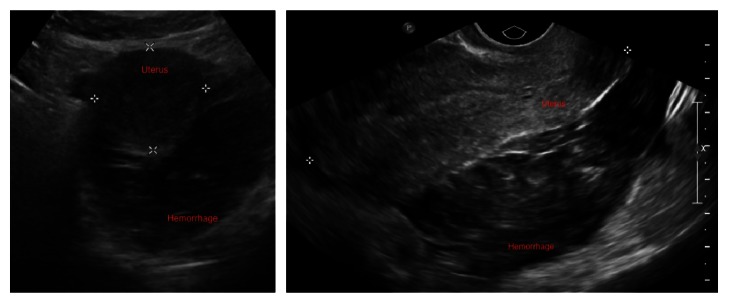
Transverse and longitudinal ultrasound images of the uterus showing intra-abdominal hemorrhage and no intrauterine pregnancy.
